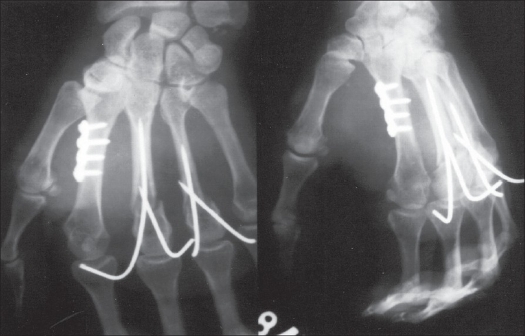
Postoperative radiographs showing good fixation with plate and screws of 2nd metacarpal fracture; and fixation with Kirschner wires for fracture neck of 3^rd^ and 4^th^ metacarpals.
